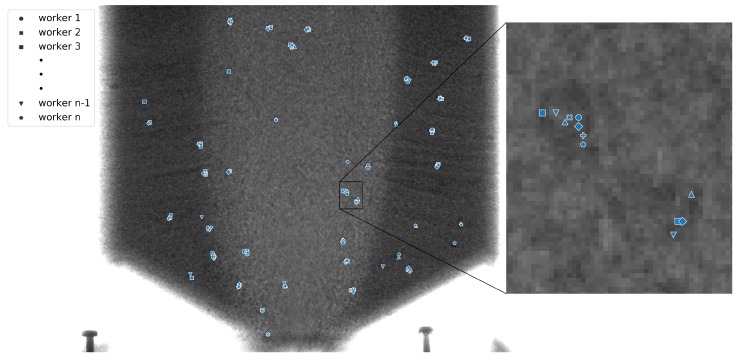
Example of the annotated X-ray image with noticeable discrepancies between the labels positioned by different workers. (Left) Full image view. (Right) Zoom-in showing slight shifts between the centers of markers set by different workers.
